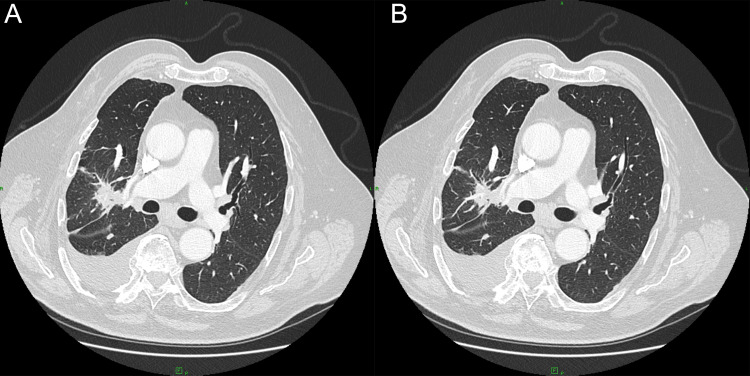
Follow-up-recent CT demonstrated residual parahilar densities without new nodules or interval progression. (A) taken in mid 2025 and (B) taken in late 2025.
